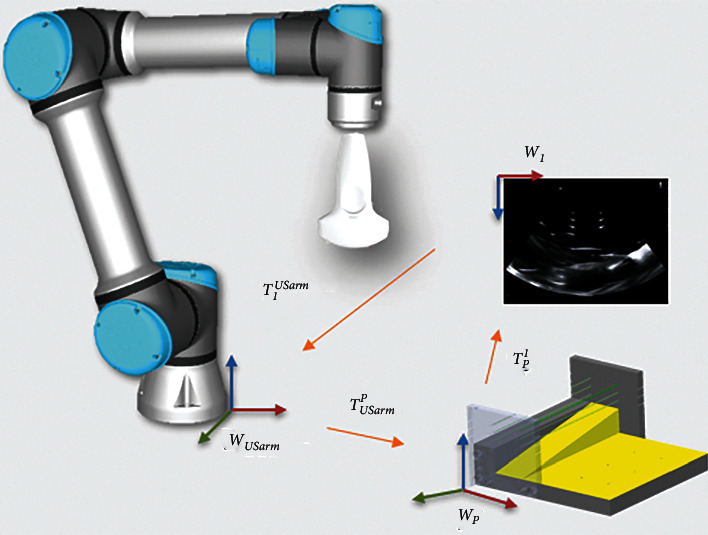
Transformations during ultrasound image calibration.
